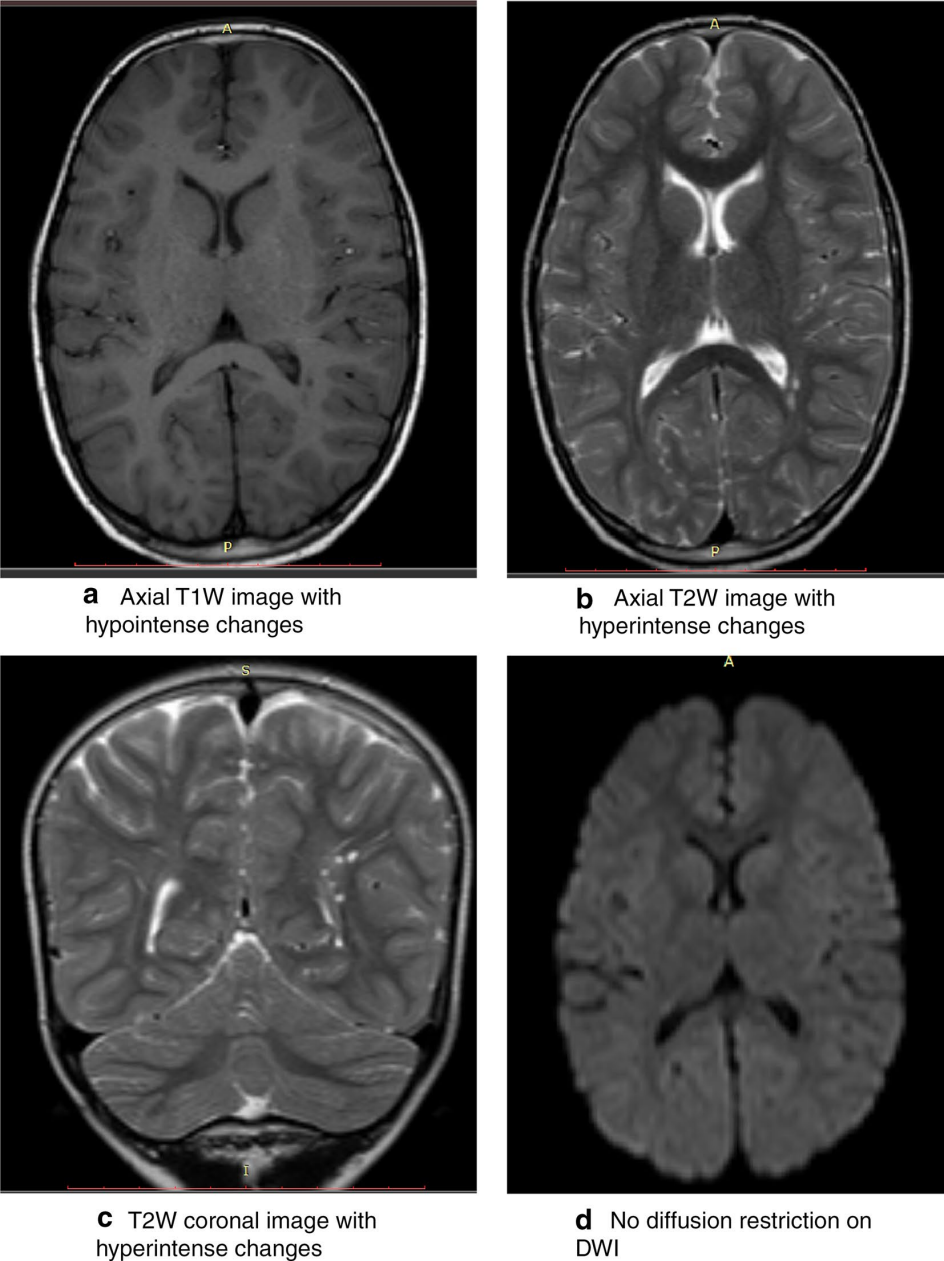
This is a set of brain MRI images of a 7 year old boy who suffered cerebral malaria at the age of 4 years. On discharge he only had hyperreflexia. His neurologic examination 3 years later was normal but the mental health assessment demonstrated oppositional defiant disorder. The brain MRI had bilateral wide spread punctate T1 weighted (T1W) hypointensities (a) and T2W hyperintensities (b) and (c) in the *white mater* and most marked in the parietal and temporal areas. There are associated with periventricular hyperintensities around the posterior horns of the lateral ventricles the largest of which measures 6 mm and widening of the sulcal spaces in the frontal and parietal lobes. The lesions exhibit no diffusion restriction on diffusion weighted imaging (DWI), (d). The changes suggest bilateral wide spread small vessel ischemia and cerebral atrophy. *T1W* T1 weighted imaging, *T2W* T2 weighted imaging, *DWI* diffusion weighted imaging
